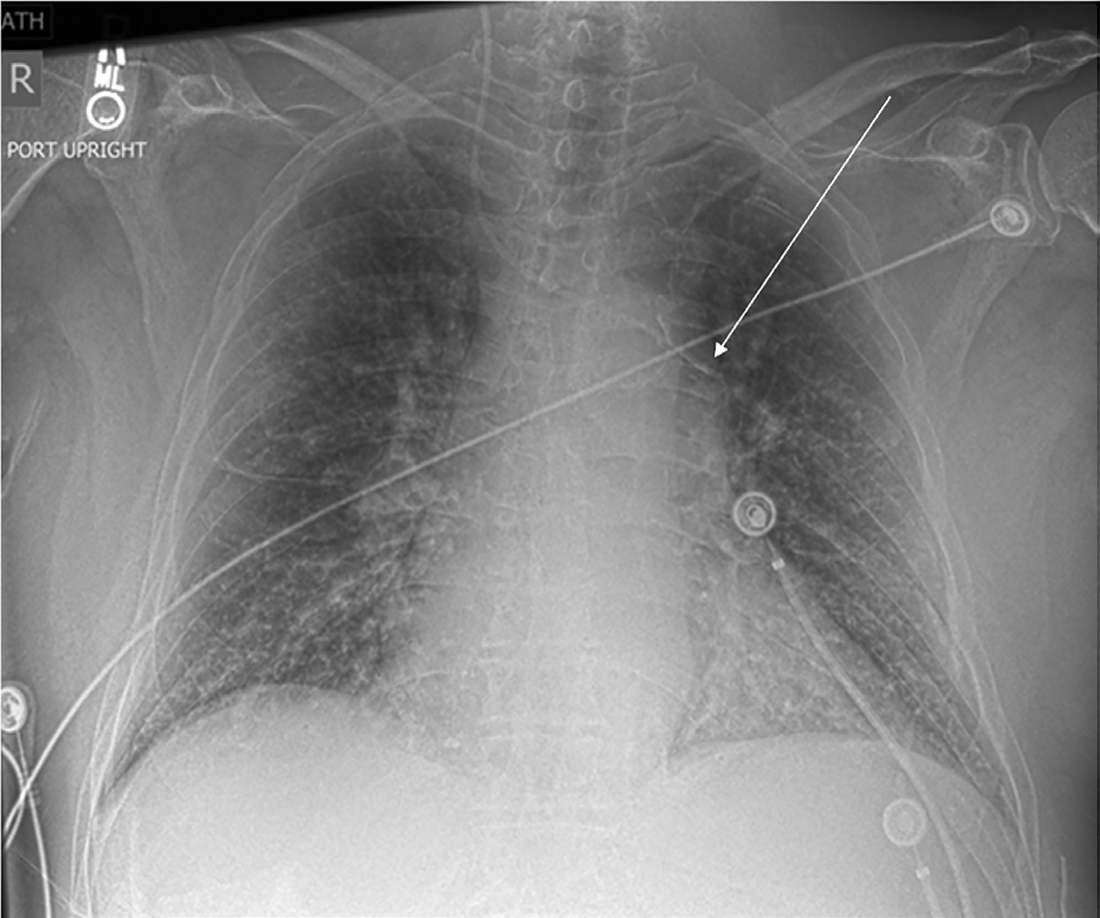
Chest radiograph obtained post right-sided internal jugular vein catheter placement, which shows catheter crossing midline right to left terminating within the left atrium (arrow).
